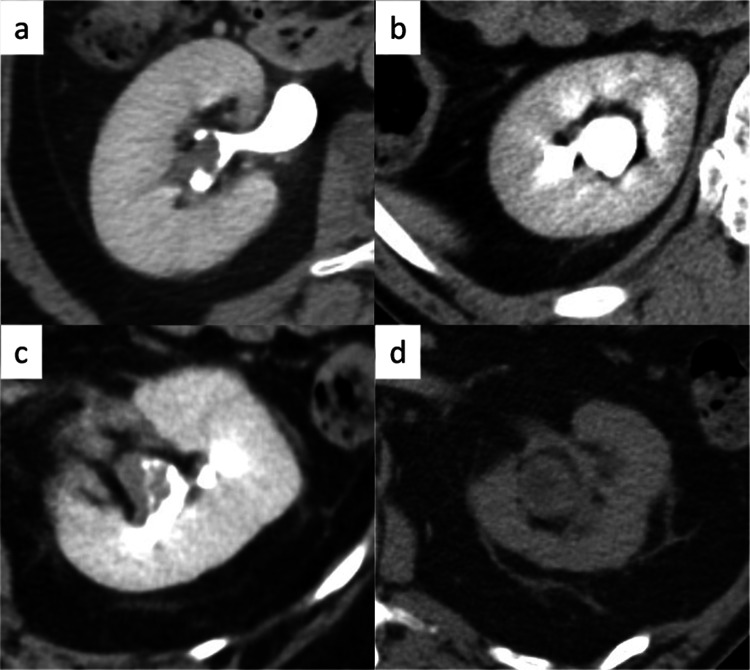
Finding of PRFS on the excretory phase of CT. The PRFS is graded according to its degree. a No PRFS. b Mild PRFS. c Moderate PRFS. d Severe PRFS. No and mild PRFS are defined as low PRFS. Moderate and severe PRFS are defined as high PRFS. *PRFS* perirenal fat stranding
